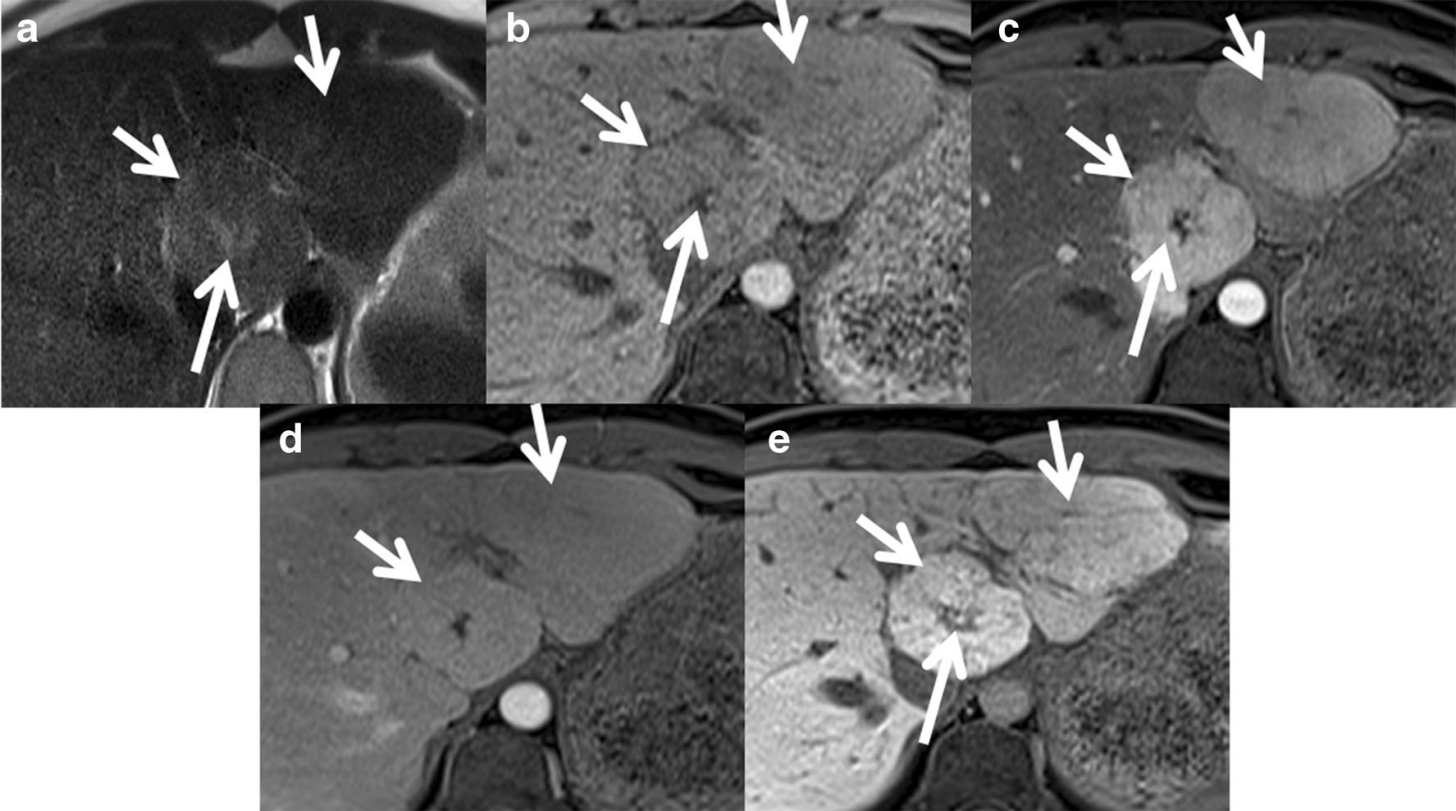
Classic FNH in a 35-year-old female with hepatobiliary-specific contrast (gadoxetate). (a) Axial T2W MRI shows two large isointense lesions in the left lobe of the liver (*short arrows*) with a T2 hyperintense central scar (*long arrow*). (b) Axial T1W MRI shows two large isointense lesions in the left lobe of the liver (*short arrows*) with a T1 hypointense central scar (*long arrow*). Axial post-contrast MRI with gadoxetate shows intense enhancement of the lesions (short *arrows*) in the arterial phase (c) becoming isointense on the portal venous phase (d) and persistent enhancement in the 20-min hepatobiliary phase (e). The central scar shows no enhancement (*long arrow*)
